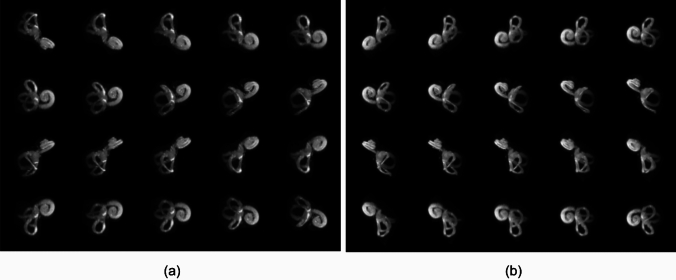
MIPs of images of the a right and b left inner ears of one subject (12,103) shown at different projection angles, where the semicircular canals connect to the cochlea acquired using 3D T2W DRIVE and dielectric pads at 7 T MRI
